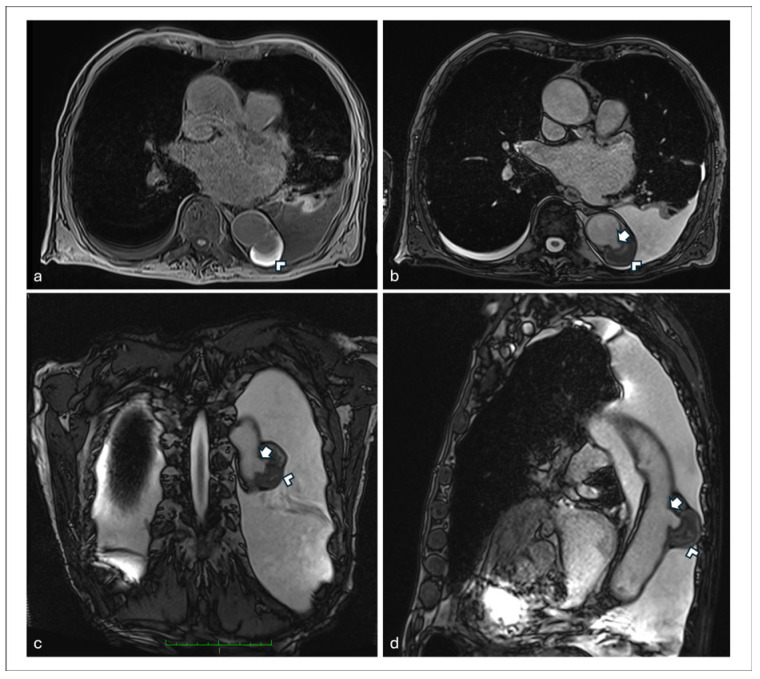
The patient was examined by a 1.5 T scanner (Siemens Magnetom Sola, Siemens Healthcare GmbH, Erlangen, Germany) and the protocol included axial and coronal T1w Dixon (3 mm slice thickness, TR 6.7 ms, TE 2.39 ms and TR 6.88 ms, TE 2.39 ms) and coronal, axial, and sagittal T2w True Fast Imaging with Steady-State Free Precession (TRUFI; 3 mm slice thickness, TR 673.53 ms, TE 2.3 ms). The MR clearly revealed the hyperintensity of the peripheral component, which was indicative of a hematoma (white arrowhead in (a)) on the T1w Dixon sequences, which then appeared hypointense in the axial, coronal, and sagittal T2 TRUFI images (white arrowhead in (b–d)); moreover, the TRUFI clearly showed the neck of the pseudoaneurysm (white arrow in (b–d)), indicating the direct communication between the lumen of the descending aorta and the lesion, allowing the diagnosis of a pseudoaneurysm. A hematoma within an aneurysm could have been considered as a differential diagnosis. Nevertheless, several features suggested that it was instead a pseudoaneurysm. Indeed, it had a disrupted calcification on the CT, a saccular outpouching rather than a fusiform shape, and it showed a narrow rather than a wide neck [1,2,3,4]. Moreover, in partially thrombosed aortic aneurysm the mural thrombus usually lines the wall, often appearing as peripheral concentric layers, while in pseudoaneurysms this often presents as a circulating sac or hematoma [1,2,3,4].
